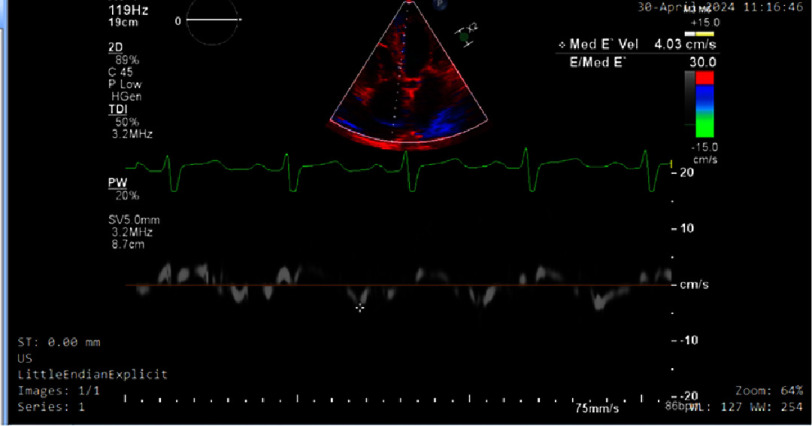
Tissue Doppler imaging (TDI) of the septal mitral annulus demonstrating an early diastolic velocity (E’) of 4.03 cm/s, yielding an elevated E/E’ ratio (>14 when combined with mitral inflow E 121 cm/s). This indicates markedly impaired myocardial relaxation and high left ventricular filling pressures, strongly supportive of grade III diastolic dysfunction in cardiac amyloidosis.
